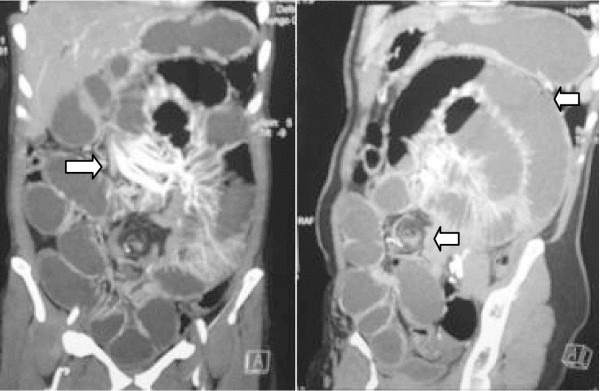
A computed tomography scan showing an aggregation of jejunal loops forming a retrocolic ‘pocket’ with a mass effect on the posterior stomach wall, a torsion zone at the root of the mesentery, and stretched and engorged mesenteric vessels.
